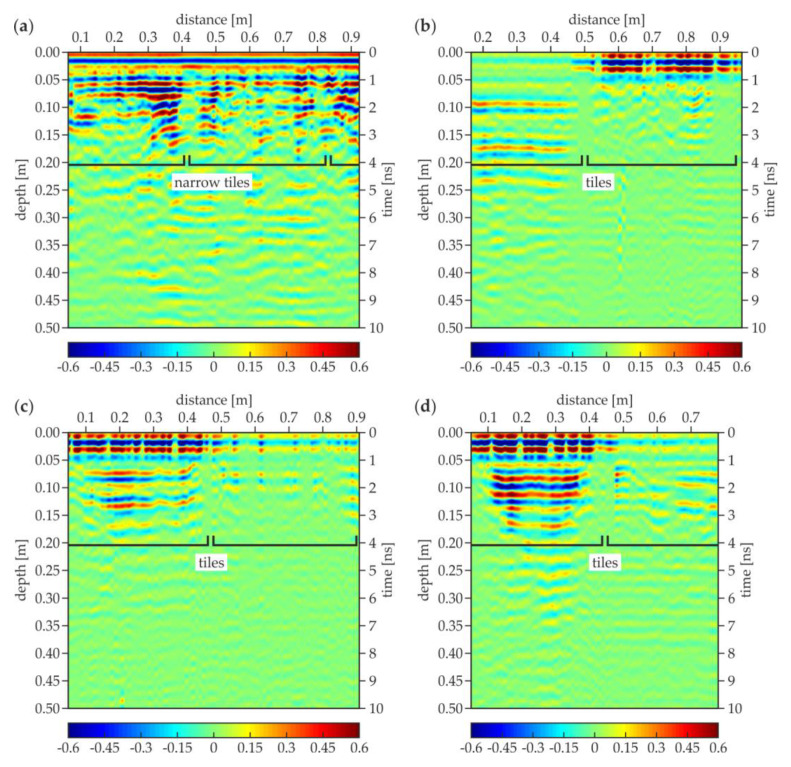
Ultrasonic B-scans for the trial pit: (a) edge 1 (trace C-1); (b) edge 2 (trace C-2); (c) edge 3 (trace C-3); (d) edge 4 (trace C-4).
